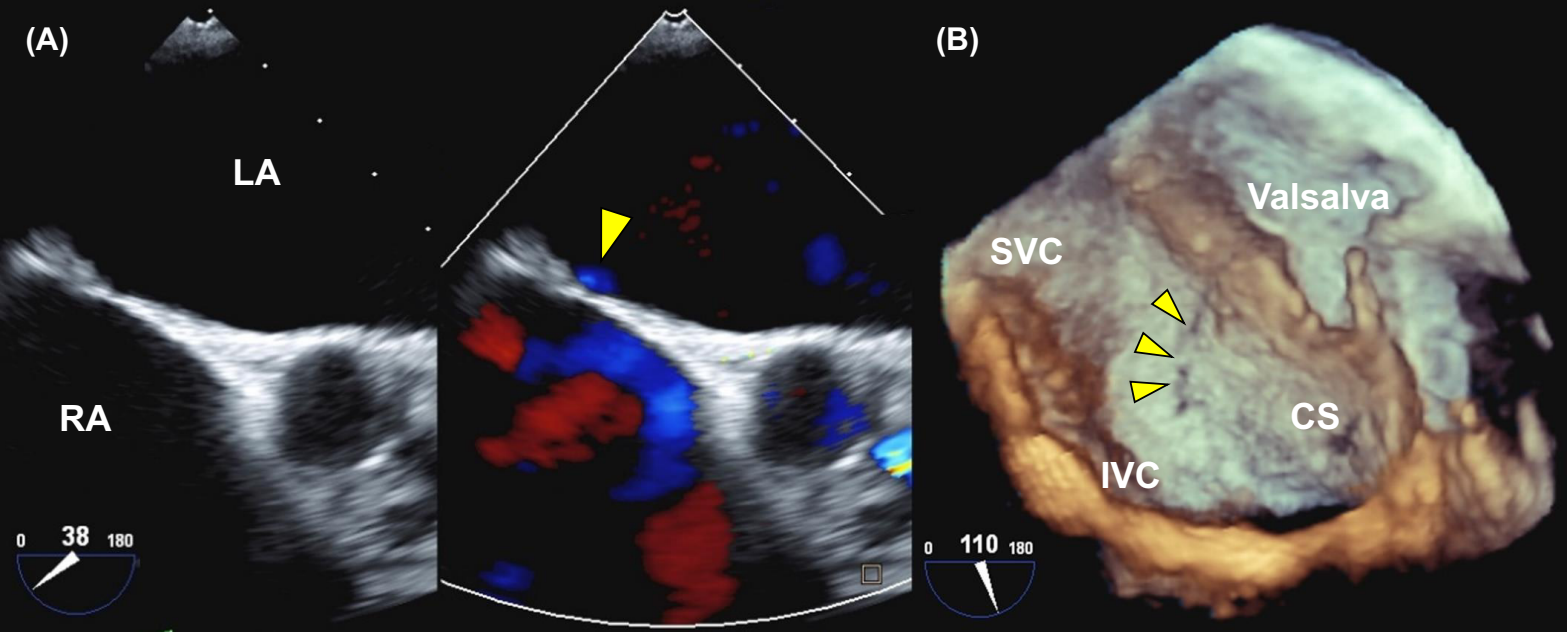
Transesophageal echocardiographic images of peri‐closure patch leakage. (A) Transesophageal echocardiography in Doppler mode detected flow from the left atrium (LA) to the right atrium (RA) through the peri‐closure patch of the ASD, suggestive of a residual shunt. (B) Three‐dimensional echography revealed a craniocaudal fistula (arrowhead) along the posterior margin of the fossa ovalis, located slightly cephalad from the opening of the coronary sinus (CS). IVC, inferior vena cava; SVC, superior vena cava.
